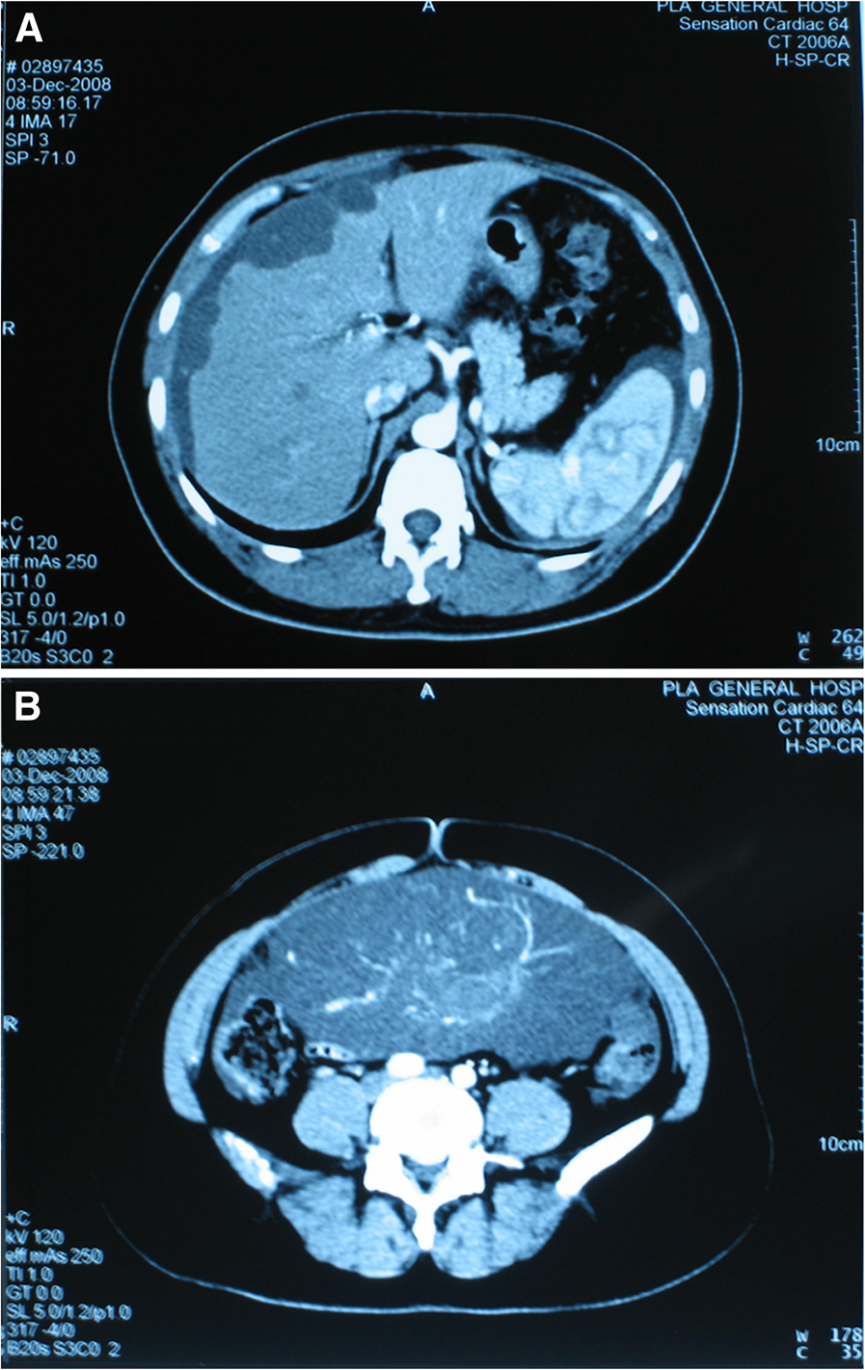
Computed tomography. (A) Multiple hepatic metastases. (B) giant local recurrent tumor infiltrating right ovary.
